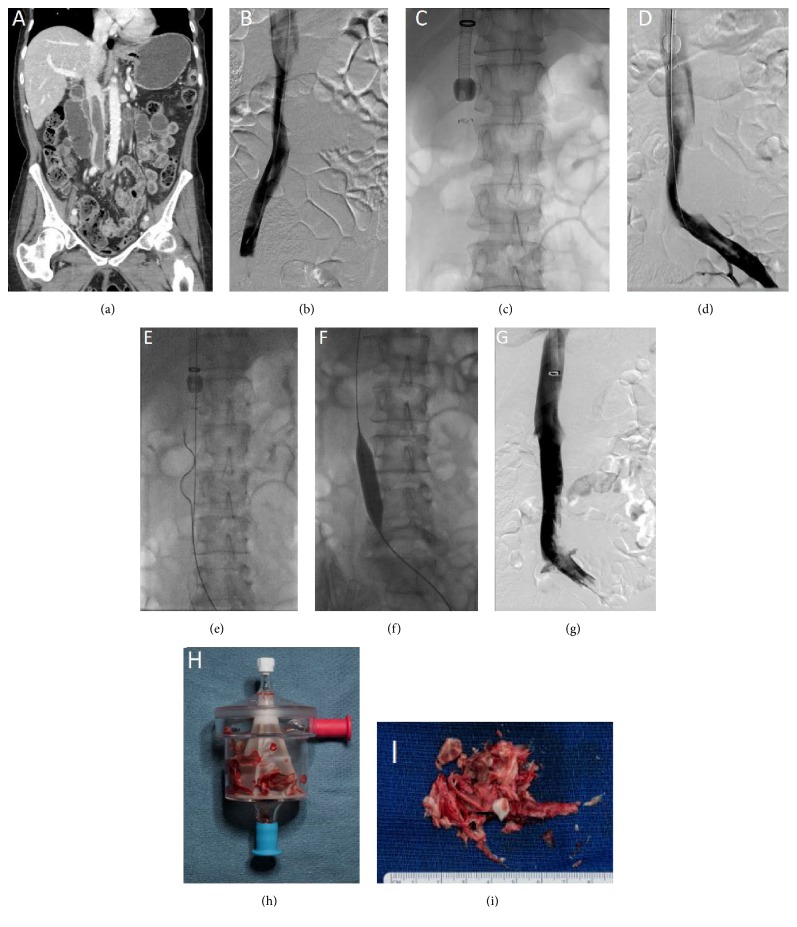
Extensive suprarenal thrombosis extending to the iliac veins treated using the AngioVac system. (a) Coronal contrast enhanced CT demonstrating the suprarenal IVC thrombosis. (b) Venogram showing the IVC thrombosis. (c) AngioVac system within the IVC engaging the IVC thrombus (d). (e) Rotational thrombectomy system is used while the AngioVac system is engaged. (f) Mechanical thrombectomy using angioplasty balloons. (g) Postprocedure venogram reveals patent infrarenal IVC and iliac veins with residual chronic thrombosis. ((h) and (i)) Aspirated predominantly chronic thrombi are shown.
